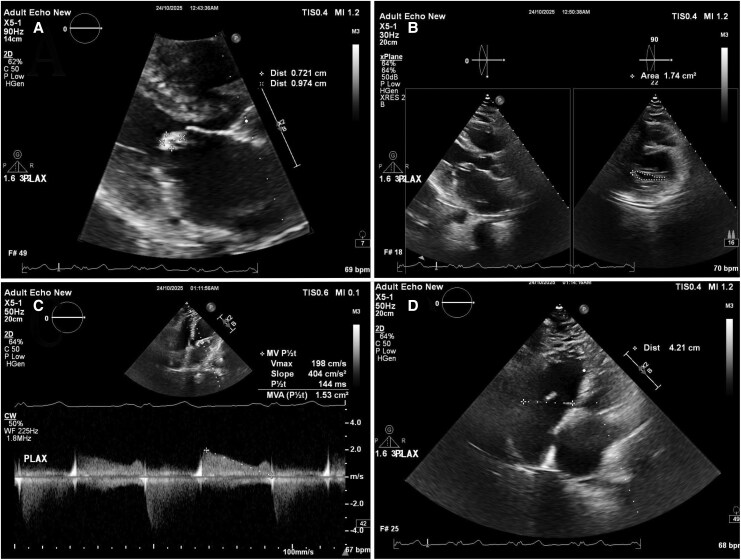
Follow-up transthoracic echocardiography (6-month follow-up). (*A*) Parasternal long-axis view demonstrating marked reduction in mitral valve mass size, measuring approximately 0.72 × 0.97 cm. (*B*) Short-axis view of the mitral valve with direct planimetry showing a mitral valve area of 1.74 cm^2^. (*C*) Continuous-wave Doppler across the mitral valve demonstrating pressure half-time (P1/2t) of 144 ms with calculated mitral valve area (MVA) of 1.53 cm^2^. (*D*) Apical four-chamber view showing right ventricular basal diameter measuring 4.21 cm, indicating improvement in right ventricular dilatation.
